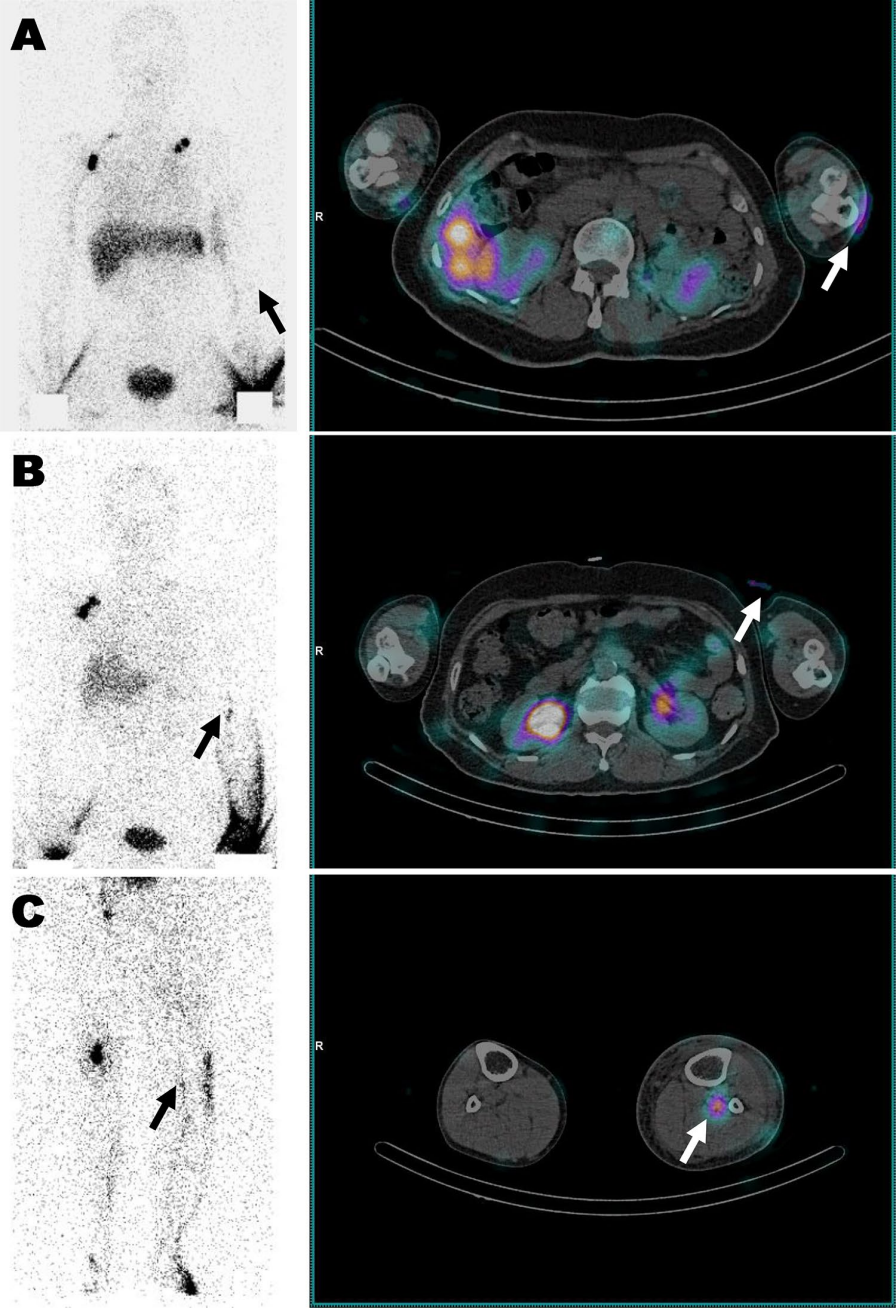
Representative cases of TLS stage change by adding SPECT/CT. Each row presents planar scintigraphy (left) and fused SPECT/CT (right) images of the same patient. The fused SPECT/CT image on the right corresponds to an axial slice at the level indicated in the planar scintigraphy on the left. (A) Modification within the stages of partial obstruction: from P-1 (DBF−, black arrow) on planar scintigraphy only to P-2 (DBF+, white arrow) on planar scintigraphy plus SPECT/CT. (B) Modification between the stages: from P-3 (intermediate LN+, black arrow) on planar scintigraphy only → T-4 (clothing contamination misinterpreted for intermediate LN, white arrow) on planar scintigraphy plus SPECT/CT. (C) Modification between the stages: from T-4 (LN−, black arrow) on planar scintigraphy only to P-3 (intermediate LN+, white arrow) on planar scintigraphy plus SPECT/CT. *TLS* Taiwan lymphoscintigraphy staging, *DBF* dermal backflow.
